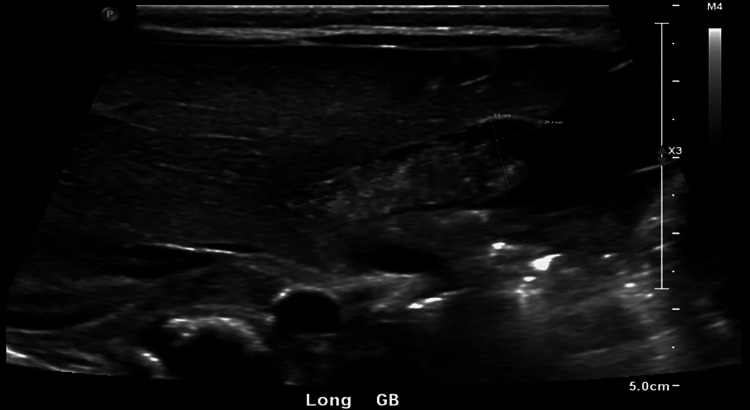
Abdominal ultrasound of cystic lesion (Case 1) Cystic lesion previously identified with intrauterine sonography seen one day post-delivery. The cystic lesion measured 4.8 x 4.7 x 2.6 cm and was observed in the left subhepatic region. The lesion was well-circumscribed with a thin wall, anechoic structure, and without demonstration of internal vascularity. The walls did not demonstrate a sonographic gut signature.
